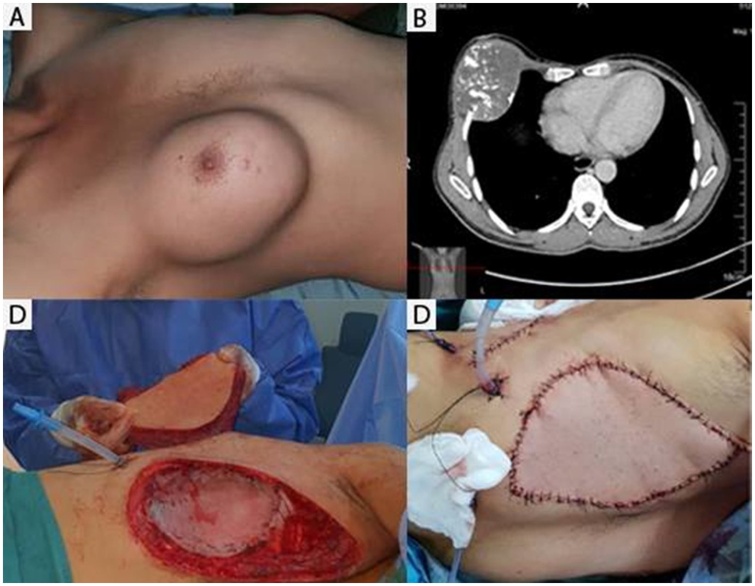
(A) Preoperative image showing the extent of the tumor B) Axial thoracic CT well- showed well-defined mass with peripheral and central calcifications measuring 07 cm × 10 cm × 11 cm. D) Reconstruction of an anterolateral chest wall defect used polypropylene plate and ipsilateral pedicle latissimus dorsi muscle flap.
